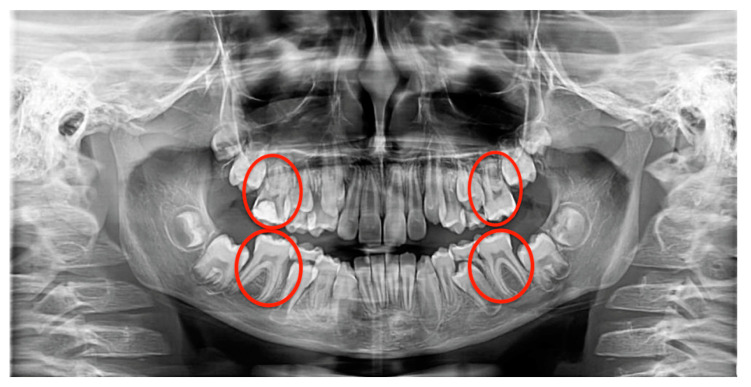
Panoramic radiograph with the first permanent molars highlighted.
